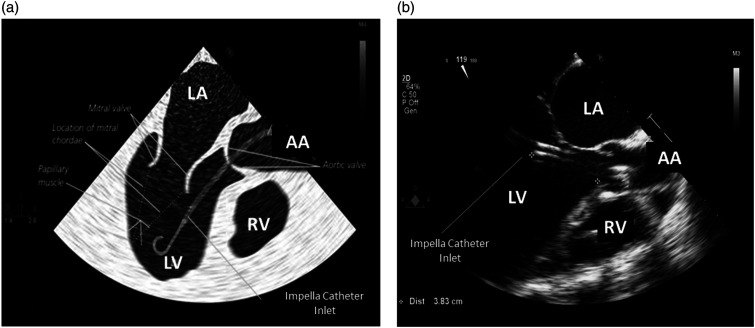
(a) Work-art representation of the ultrasound anatomy of a transesophageal mid-esophageal long-axis view (120°) (courtesy of Abiomed, Danvers, MA, USA); (b) Transesophageal mid-esophageal long-axis view (120°) of an Impella CP positioned at 3.83 cm from the aortic annulus. RV: right ventricle, LV: left ventricle; LA: left atrium; AA: ascending Aorta.
